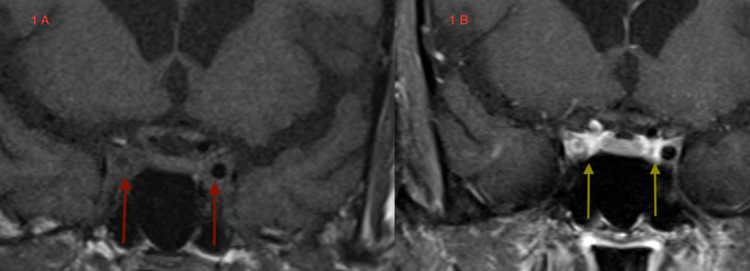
Coronal T1-weighted MRI images. 1A - unenhanced images show carotid siphon arteries in cavernous sinuses (red arrows). 1B - gadolinium enhancement shows intensely enhancing cavernous sinus medial walls (yellow arrows) with luminal narrowing of right carotid siphon. MRI: Magnetic Resonance Imaging
